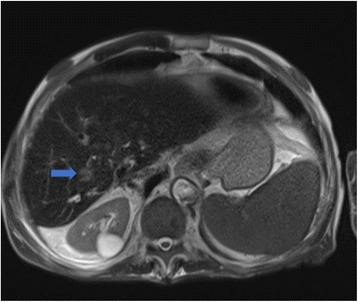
MRI investigation of the liver as described in Fig. 2. A third nodule (1.2 cm diameter) was described in the right liver lobe (arrow)
